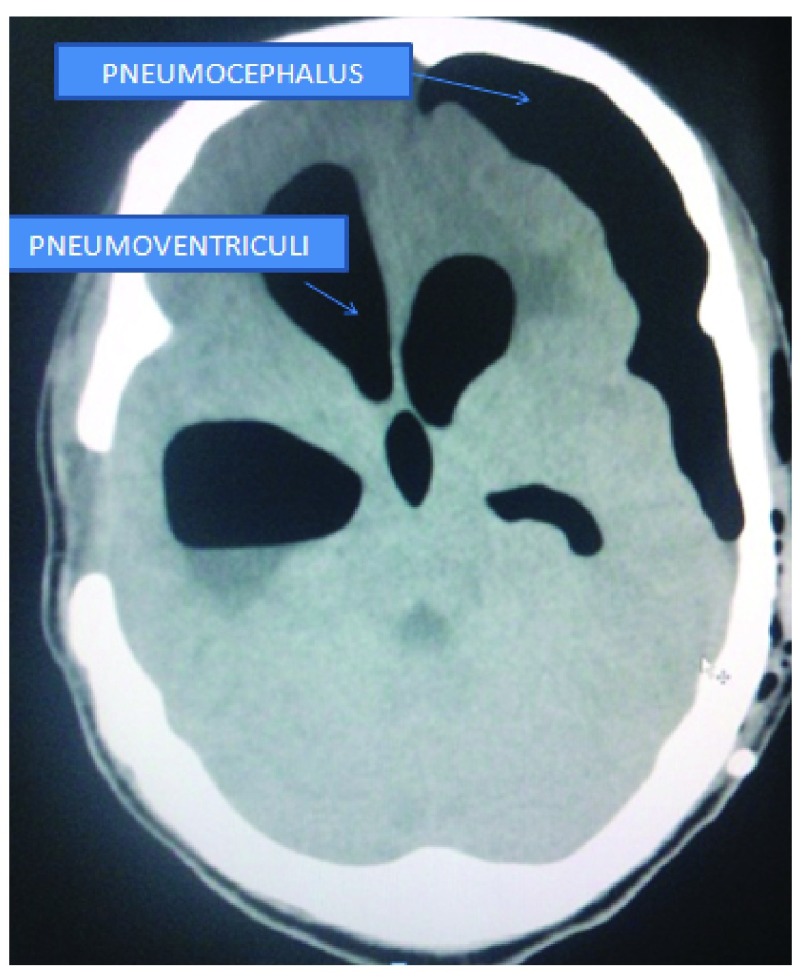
CT image showing presence of pneumocephalus and pneumoventriculi.
